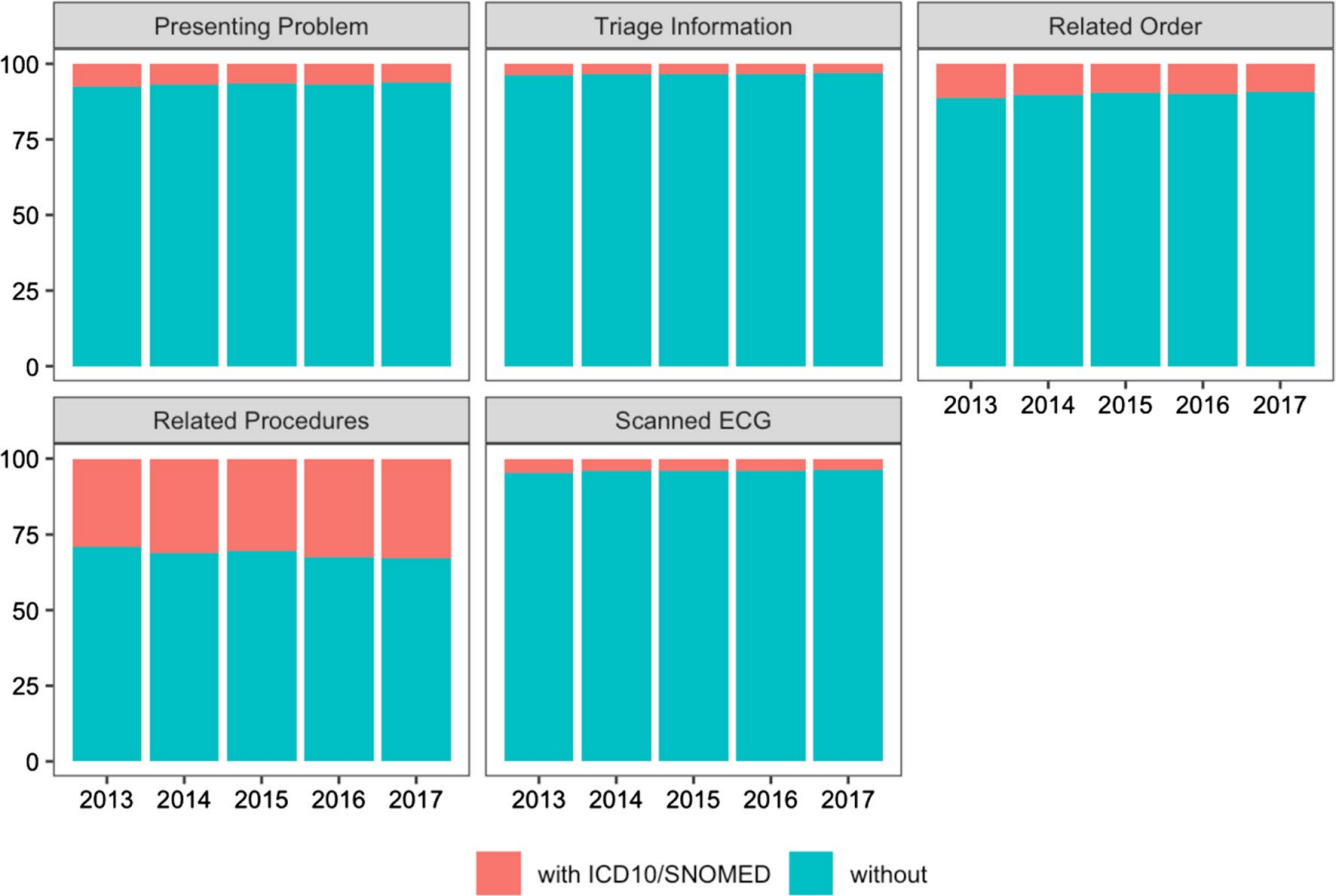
Percentage of eligible encounters with diagnostic codes (ICD-10/SNOMED CT) for each inclusion criteria, by year
